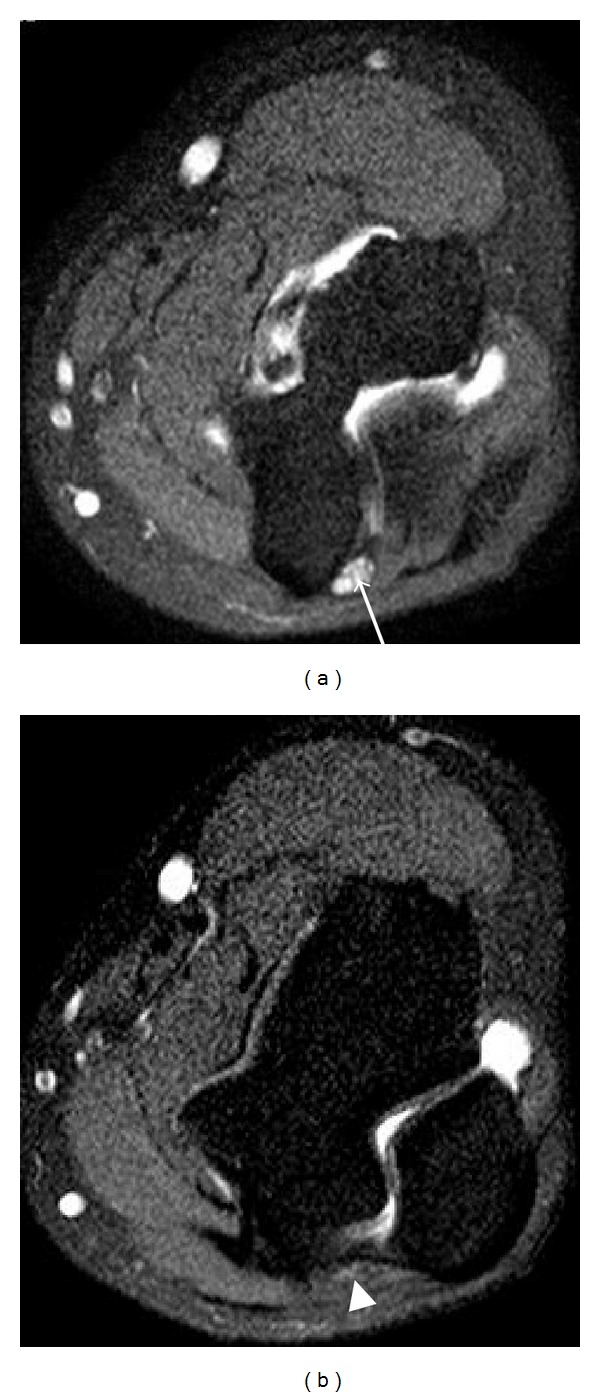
A 17-year-old patient suffered right cubital tunnel syndrome. MR arthrogram of the elbow was performed to evaluate for loose body. Axial T2-weighted with fat-saturated (a, b) images reveal swollen and edematous ulnar nerve (arrow) at the level of proximal cubital tunnel, and normal size distally (arrowhead).
